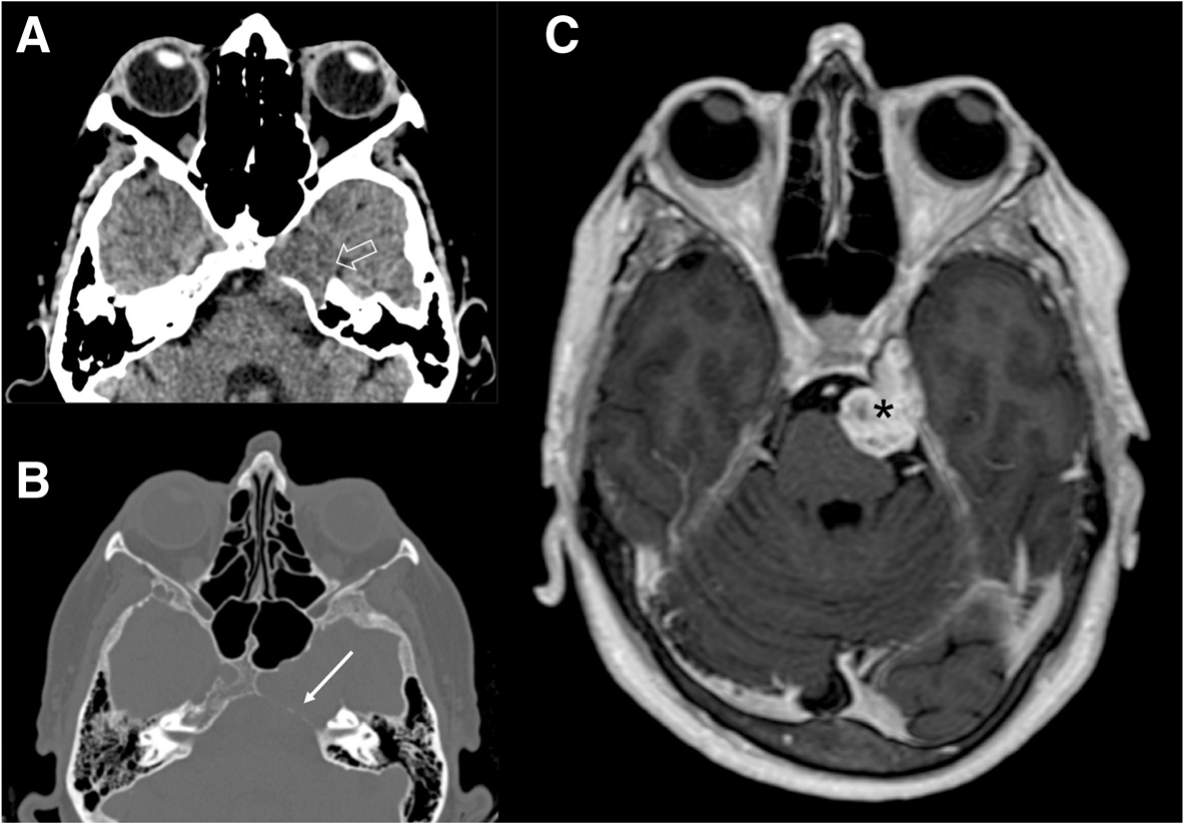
Large trigeminal schwannoma. CT axial image (soft tissues and bone windows, a, b, respectively) demonstrates a mild iso-attenuated mass (empty arrow) with erosion of the left petrous apex (arrow); MRI T1-weighted post-gadolinium axial image (c) shows an avid enhancing dumbbell-shaped mass (asterisk), that extends in the Meckel’s cave, along the cisternal course of the left trigeminal nerve
